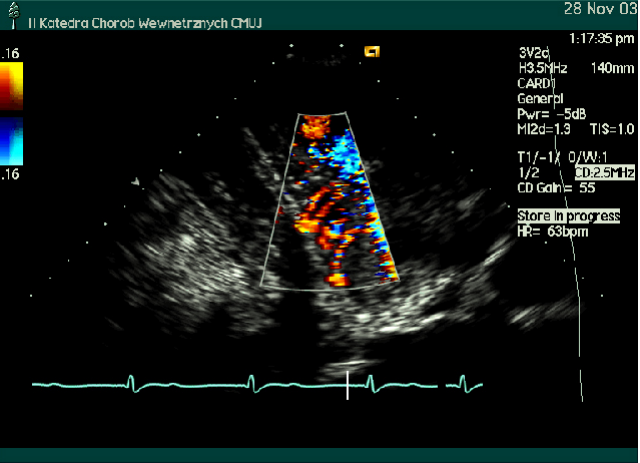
Modified apical 2-chamber view, middle segment of the inferior wall on color Doppler examination. Small (arch-shaped) arteries branching of the right posterior descending (RPD) coronary artery are seen.
